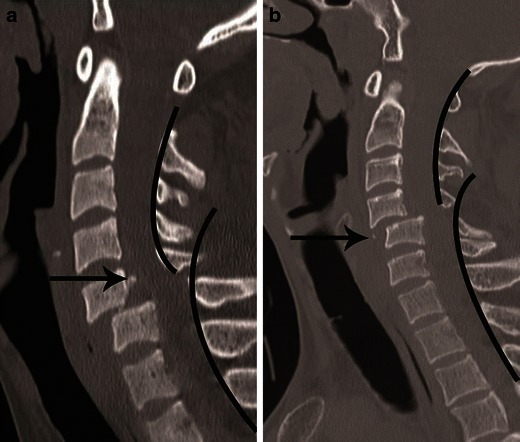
Bilateral versus unilateral facet joint dislocation. a Mid-sagittal CT image shows anterior translation of C5 over C6 by more than 50 % anteroposterior width of the C6, highly suggestive of bilateral facet joint dislocation, which was confirmed on parasagittal images (not shown). Large triangular bony fragment is noted at the posteroinferior corner of C5 (*arrow*) with diffuse loss of C5–C6 intervertebral disc space. b Mid sagittal CT image shows anterior translation of C4 over C5 by less than 50 % anteroposterior width of C5 (approximately 25 %), highly suggestive of unilateral facet joint dislocation, which was confirmed on parasagittal images (not shown). Small triangular bony fragment is noted at the anteroinferior corner of C4 (*arrow*) with loss of C4–C5 disc space. Disruption of spinolaminar line (*black curved lines*) at the level of anterolisthesis with anterior displacement of the same vertebra and lamina (C5 in a and C4 in b) is highly suggestive of hyperflexion injury. Presence of small avulsion fragments at the discovertebral junction with loss of intervertebral disc height is associated with disc injury, which needs further evaluation with MRI
